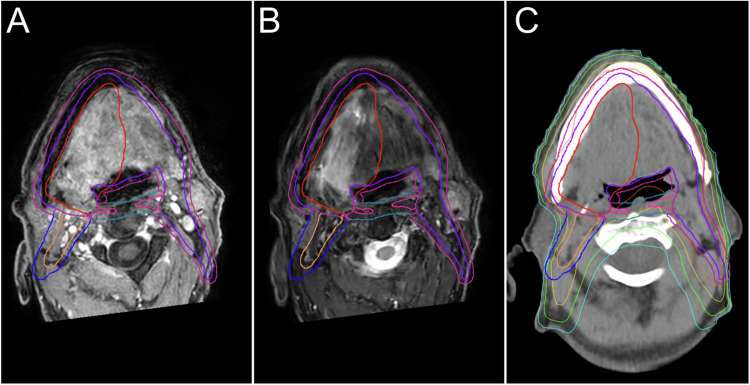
Contrast-enhanced fat-saturated T1w (A), STIR-weighted images (B), and related centering CT (C) with the superimposed distribution of doses, GTV (red line), and PTV (blue line) in a patient affected by oral floor squamous cell carcinoma
